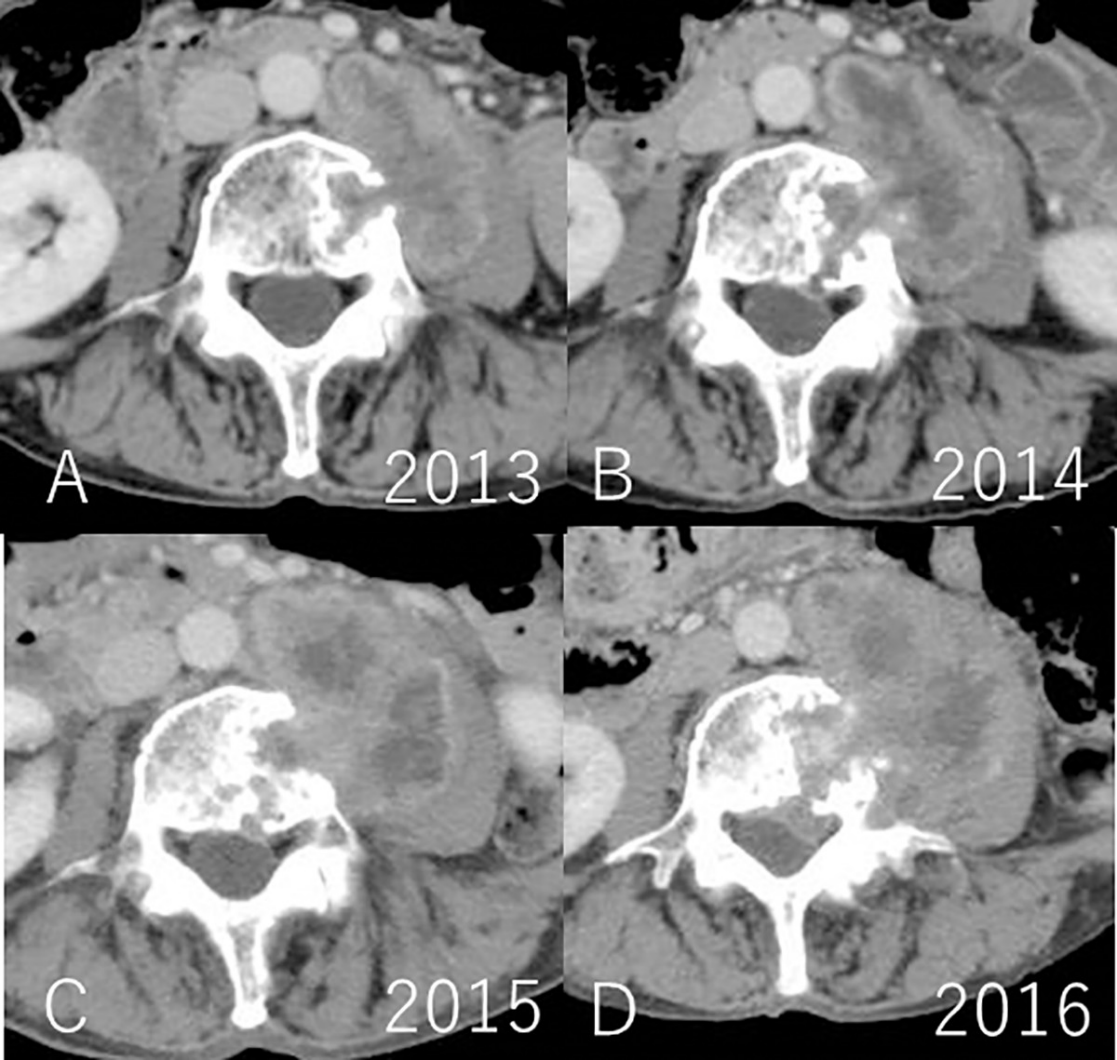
CT scan following contrast injection in 2013; B. CT scan following contrast injection e in 2014; C. CT scan following contrast injection in 2015; D. CT scan following contrast injection in 2016 (Case no.7). Base line diameter of the tumor was 57 mm, and final diameter of the tumor was 83 mm. Finally the tumor was surgically removed with bone graft and spinal instrumentation.
